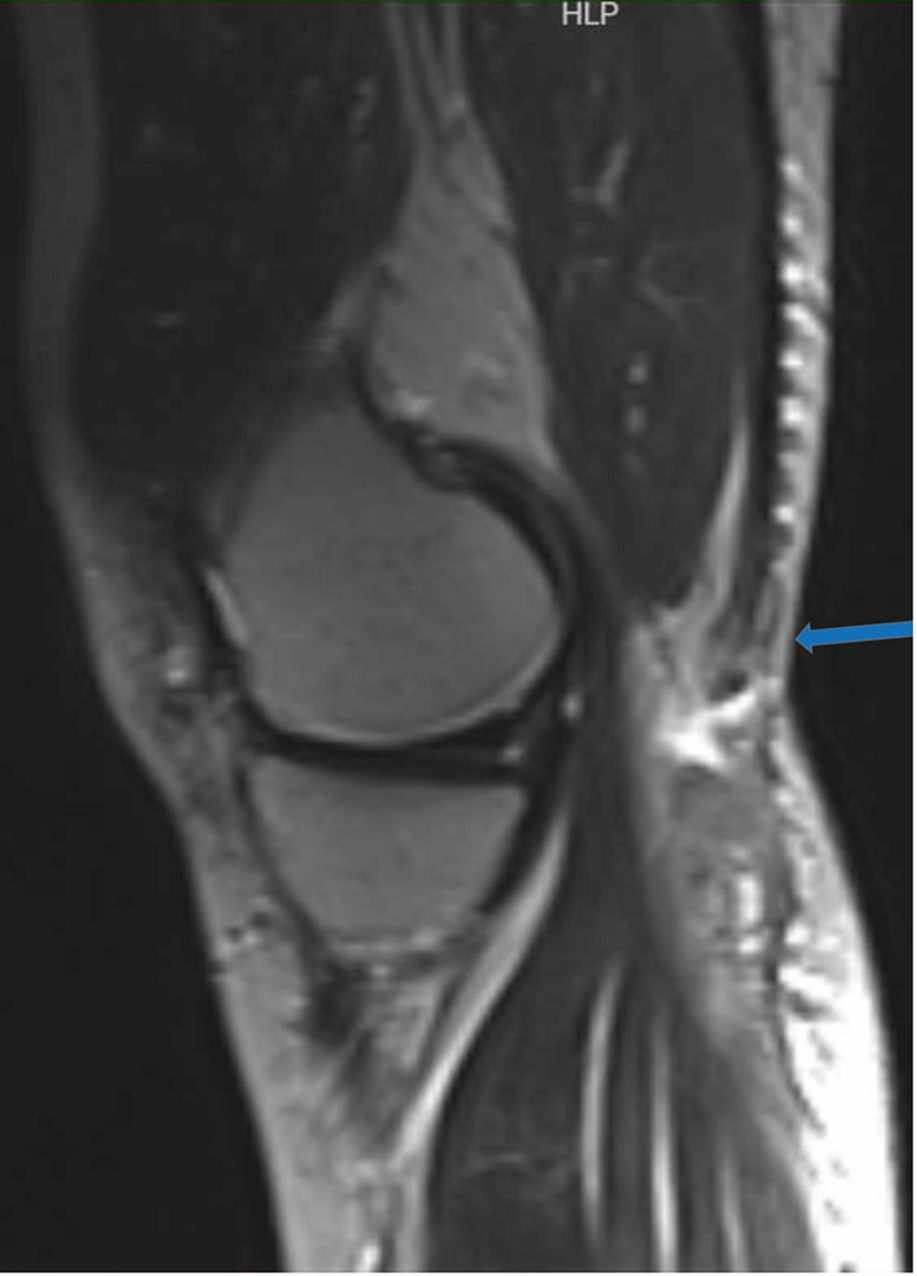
Sagittal T2 magnetic resonance imaging. The left knee joint shows a rupture of the semitendinosus tendon (blue arrow) with distal fluid collection.
